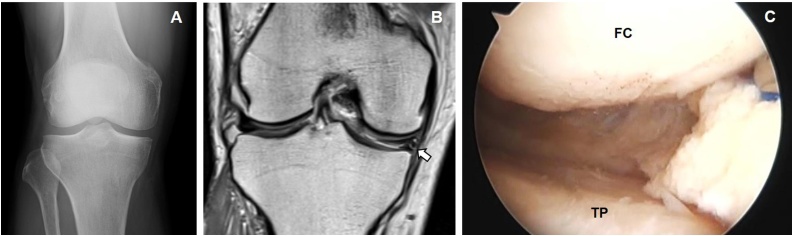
(A) Initial anteroposterior radiograph showed no significant findings. (B) T2-weighted magnetic resonance images showed a straight high intensity line indicating a horizontal tear of the medial meniscus (red arrow). (C) Intraoperative image of arthroscopic meniscectomy. Meniscus height is obviously uneven in between the middle portion of the meniscus and the resected posterior portion.
